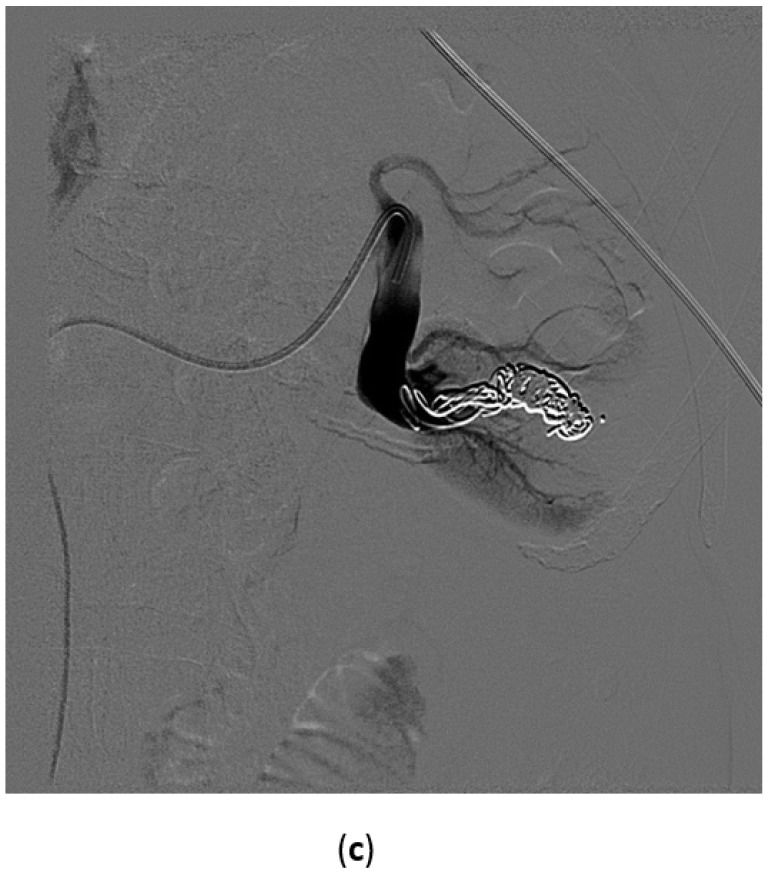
Arteriovenous fistula 59-year-old female, occasional finding on US examination. The patient had no history of previous trauma or renal intervention; thus, the AV fistula was supposed to be congenital. (a) Contrast-enhanced CT (arterial phase). The coronal plane well shows dilated vascular structures in the inferior half of the left kidney; (b) Selective angiogram of the left kidney confirms the AV fistula with huge dilatation of the renal vein; (c) Disappearance of the fistula after embolization with several detachable coils.
